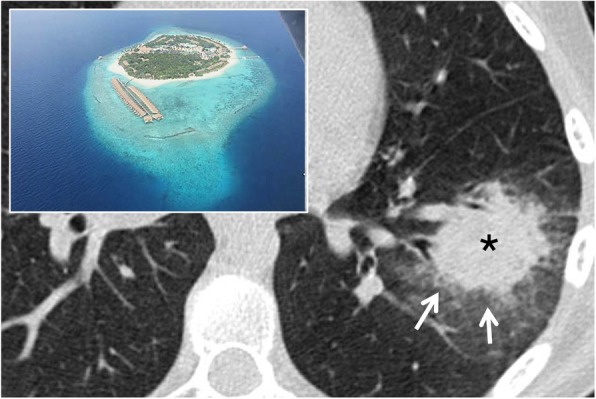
Halo sign. The CT image shows the “halo sign” in a patient with angioinvasive aspergillosis: in the left lower lobe, it is possible to appreciate a round consolidation (black asterisk) with peripheral ground glass (white arrows), which suggests a hemorrhagic process. This ring or peripheral ground glass is similar to the sea’s appearance adjacent to an atoll
